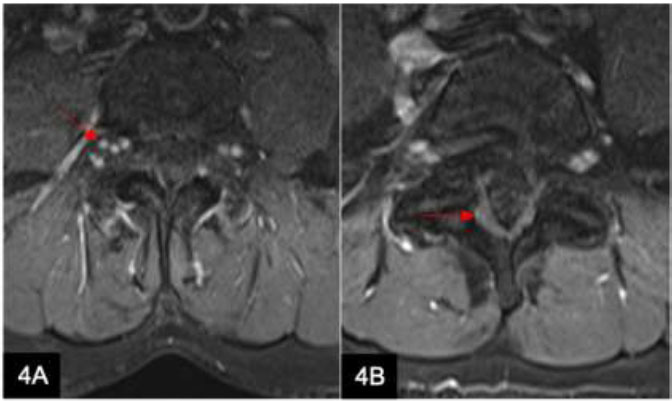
A 53-year-old woman with degenerative disc disease presents with radiculopathy. Axial T1-weighted (w) post-contrast (A) MRI lumbar spine image shows multiple nodular enhancements along the exiting nerve roots at the L3-L4 level, in keeping with multiple schwannomas (arrow). Axial T1w post-contrast (B) image shows leptomeningeal enhancement of the cauda equina (arrow).
